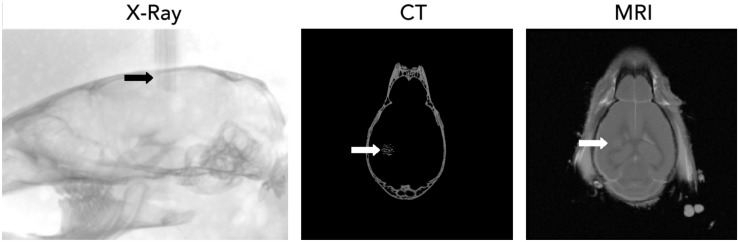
Localization of CHIME microwire bundles using X-ray computer tomography (CT, left: individual X-ray projection) and magnetic resonance imaging (MRI).
